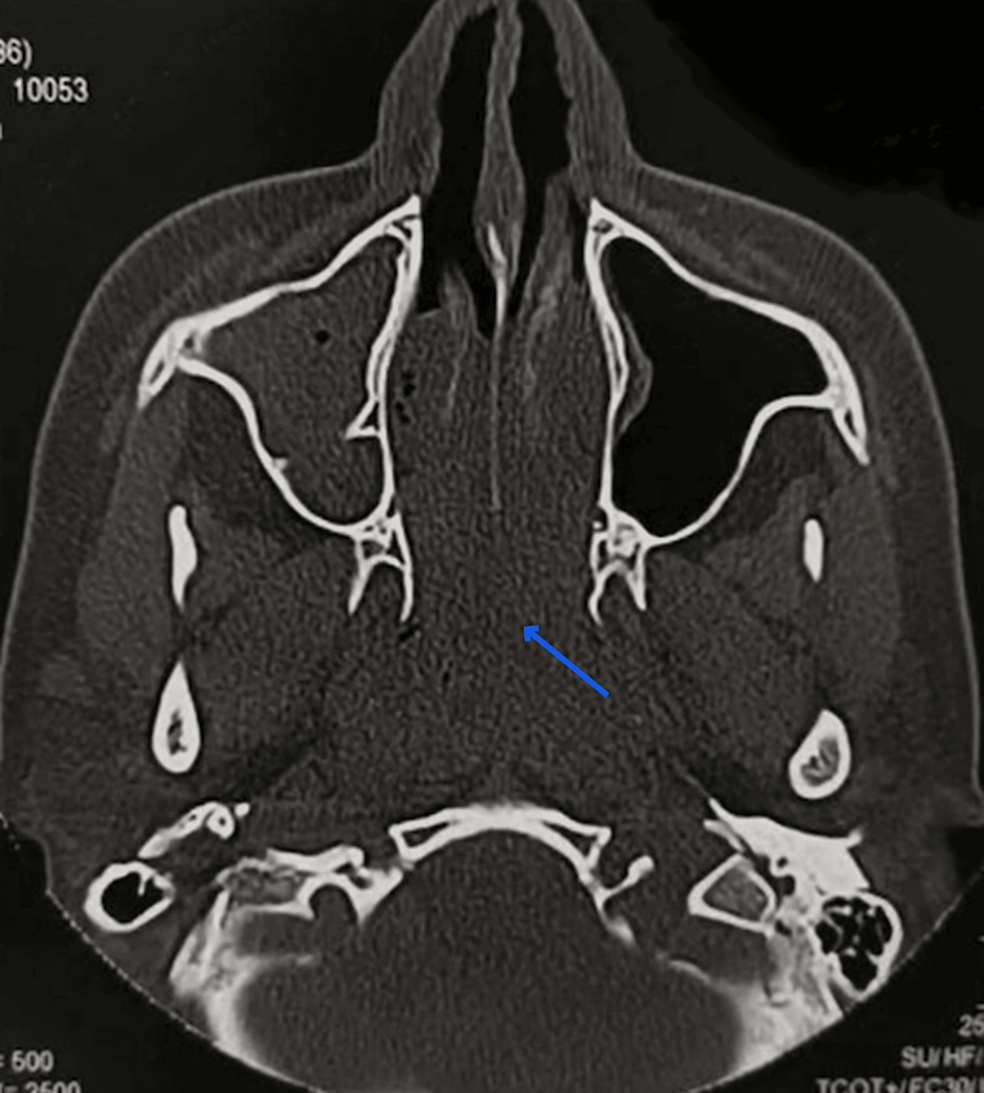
CT scan findings. The paranasal sinus shows right-sided maxillary sinusitis and soft tissue mass filling the bilateral inferior meatus and extending posteriorly into the nasopharynx. The blue arrow points toward the mass occupying the bilateral inferior meatus and extending into the nasopharynx.
